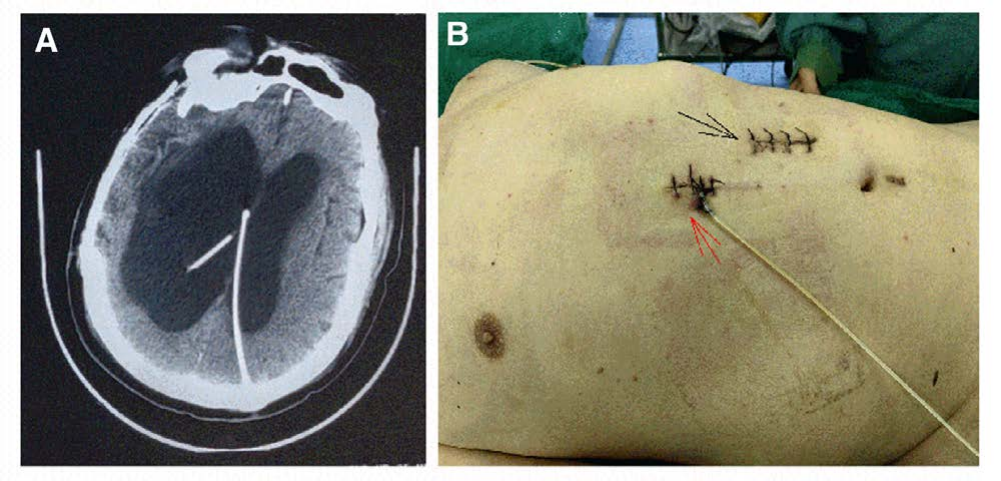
(A) Brain CT showing hydrocephalus with changes observed after bilateral ventricular shunt placement. (B) After adjustment of the peritoneal end of the shunt tube in both ventricles, the shunt on the left side of the abdomen was not explored (black arrows), and the right ventricular drain was externally drained (red arrows). CT = computed tomography.
